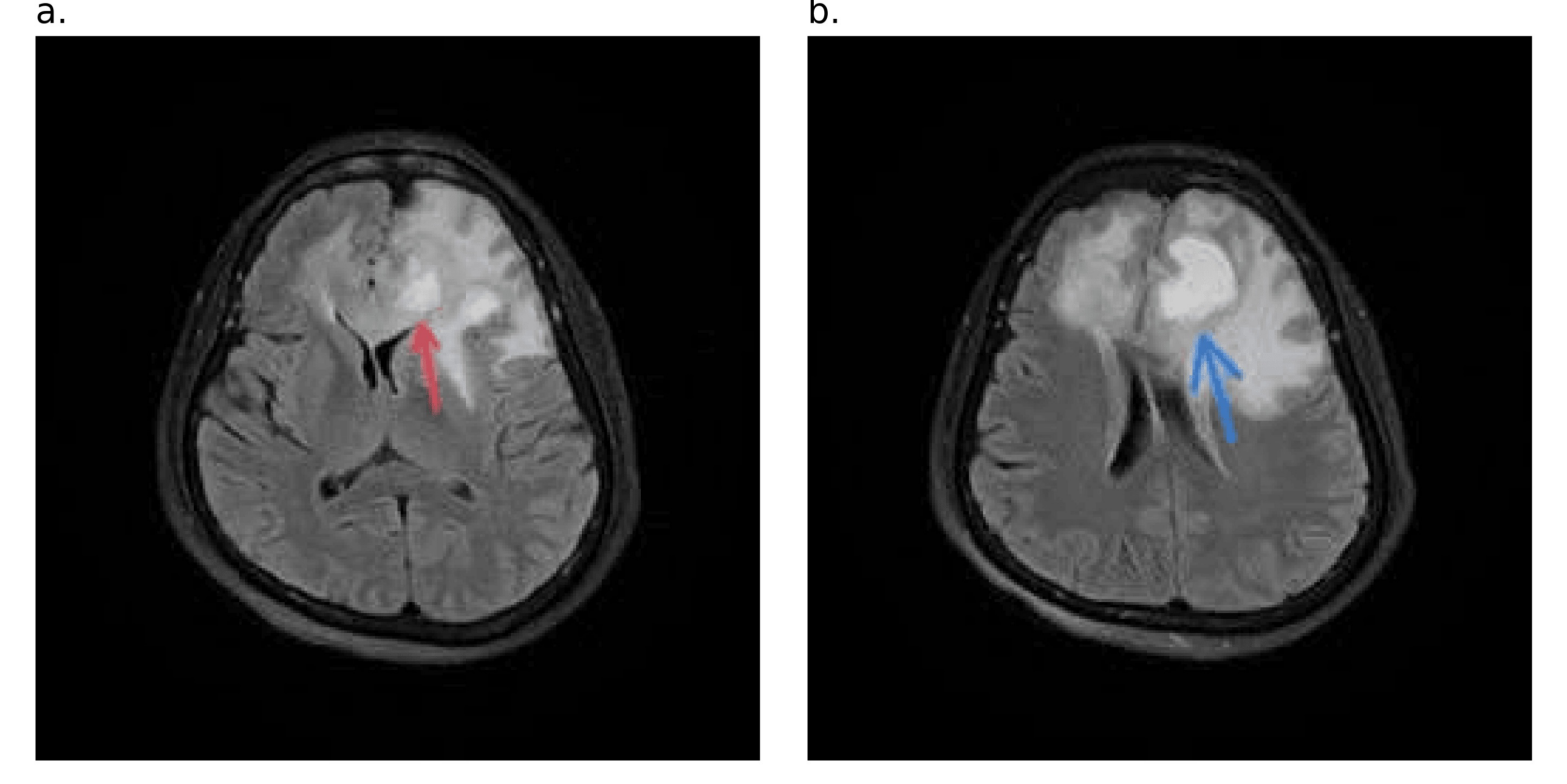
Axial FLAIR MRI image a. Peripherally enhancing tumor (red arrow) at the level of the capsuloganglionic region, and b. (blue arrow) at the level of the body of the lateral ventricles FLAIR: fluid-attenuated inversion recovery; MRI: magnetic resonance imaging
